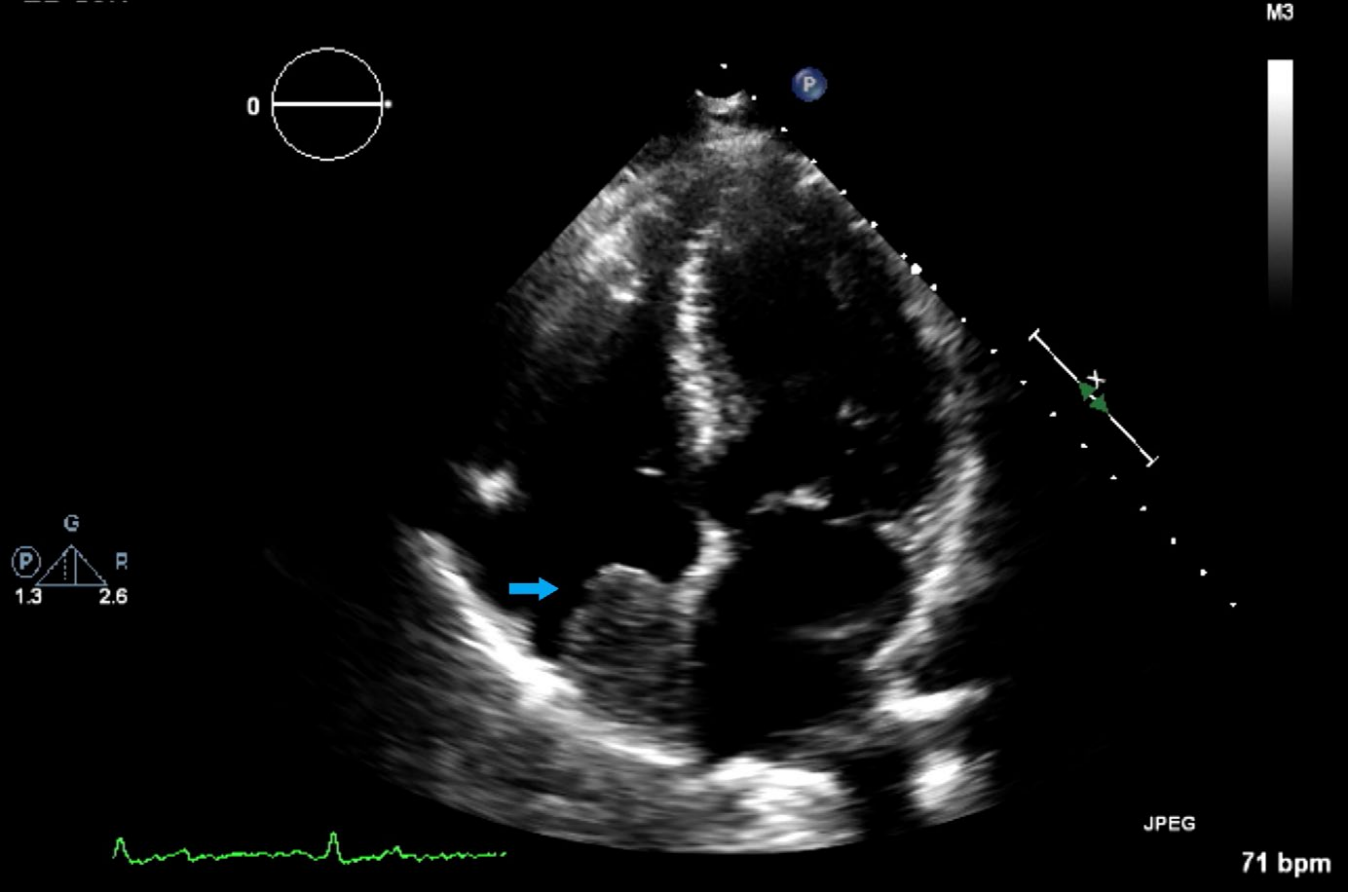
Arrow showing cardiac lipoma in the right atrium on transthoracic echocardiogram
